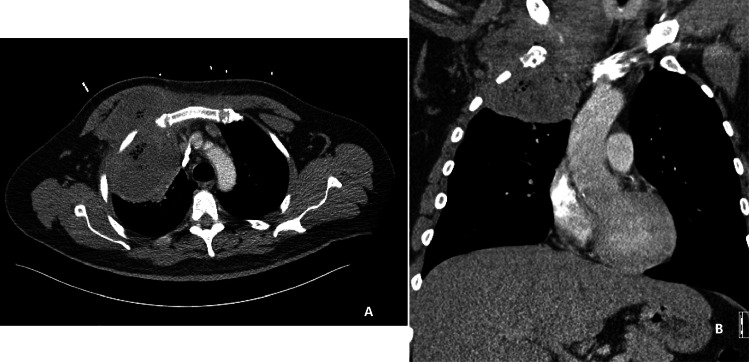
CT scan chest with empyema necessitans. (A) Axial, (B) Coronal: 48 yr M with uncontrolled DM showing large medium-density peripherally enhancing infected hematoma centered around a fractured right first rib wall with intrathoracic and chest wall components. Findings are most compatible large right-sided empyema secondary to infected intrapleural hematoma found to be tracking to skin with internal air representing necrosis consistent with empyema necessitans. The patient underwent chest wall exploration and pleural space drainage for optimization of drainage. Images Courtesy by Dr. Ory Wiesel
